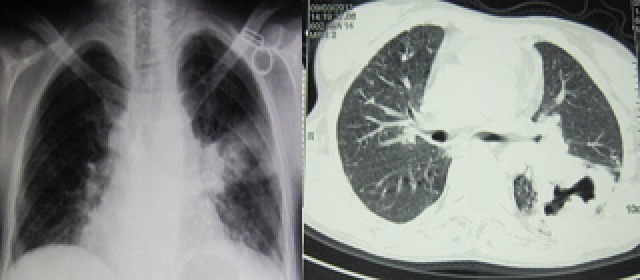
Radiographie et scanner thoraciques d'une patiente de 40 ans montrant une PT du lobe inferieur gauche
